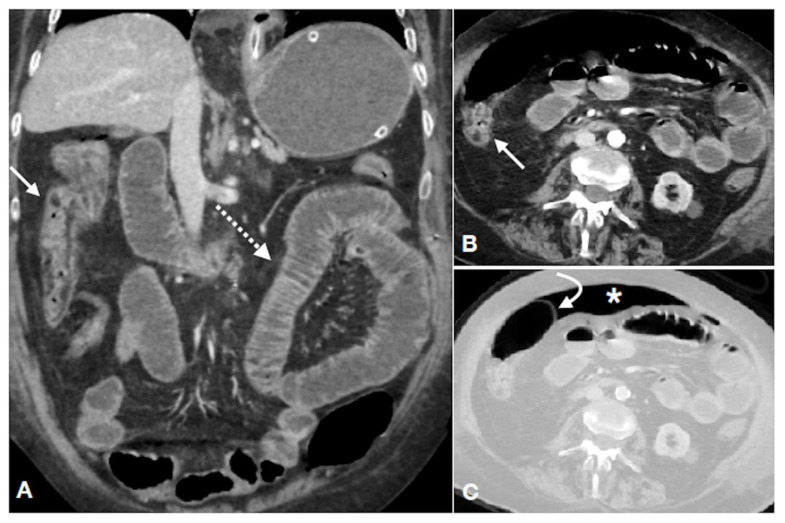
CT of an 85-year-old female with pelvis fracture, complaining of abdominal pain and constipation. Enhanced CT in portal-venous phase, coronal (A) and axial (B,C) views, showing thickening of the ascending colonic wall with mucosal hyperemia and submucosal edema ((A,B) arrow) and increased enhancement of the small bowel wall ((A), dotted arrow); these are findings of small and large bowel reperfusion after ischemia. The colonic wall is partly thickened ((B), straight arrow), due to reperfusion phenomena, and partly thinned and distended ((C), curved arrow), due to ischemia without reperfusion, leading to colonic perforation with peritoneal free air ((C), asterisk).
